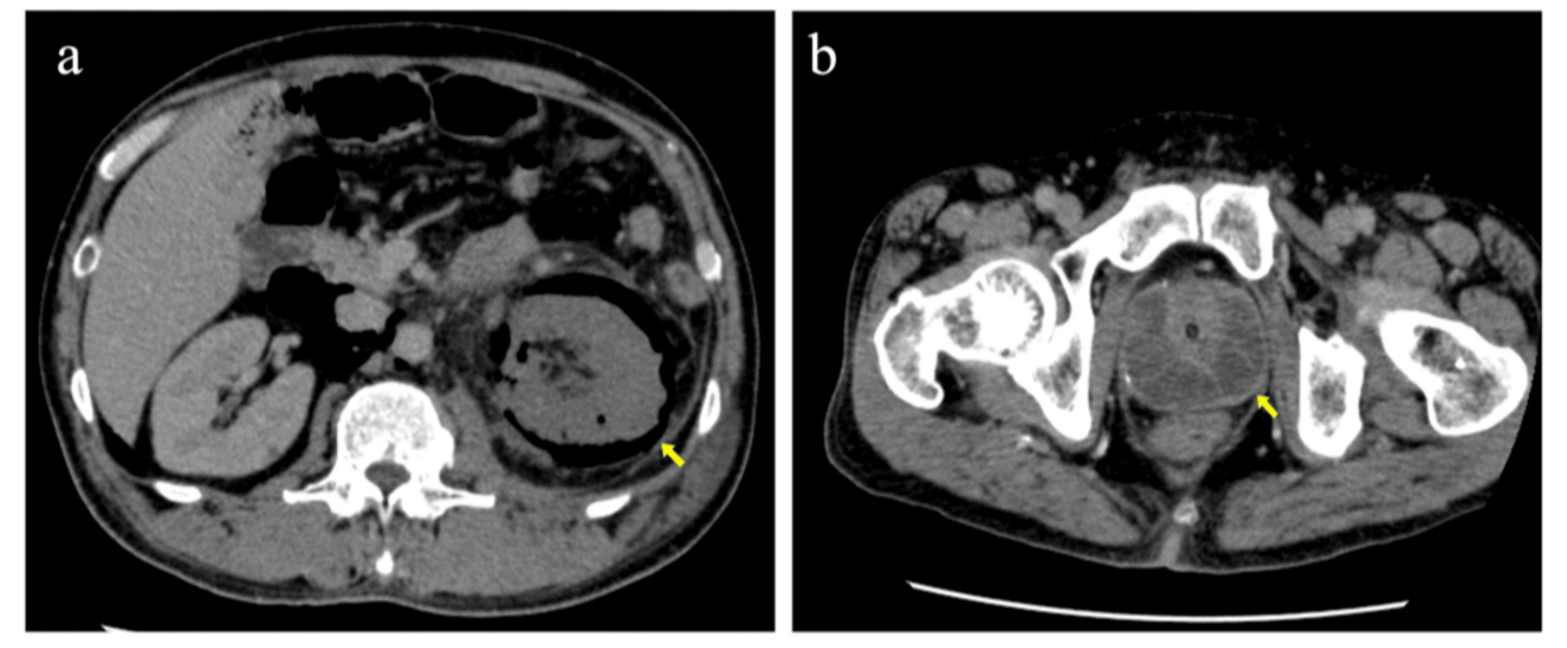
Contrast-enhanced computed tomography findings on admission. (a) Contrast-enhanced axial computed tomography on admission demonstrating extensive gas formation in the left renal parenchyma and perirenal space (yellow arrows). (b) Contrast-enhanced axial computed tomography demonstrating multiple low-density lesions with internal septations in the prostate gland (yellow arrow), consistent with a prostatic abscess.
